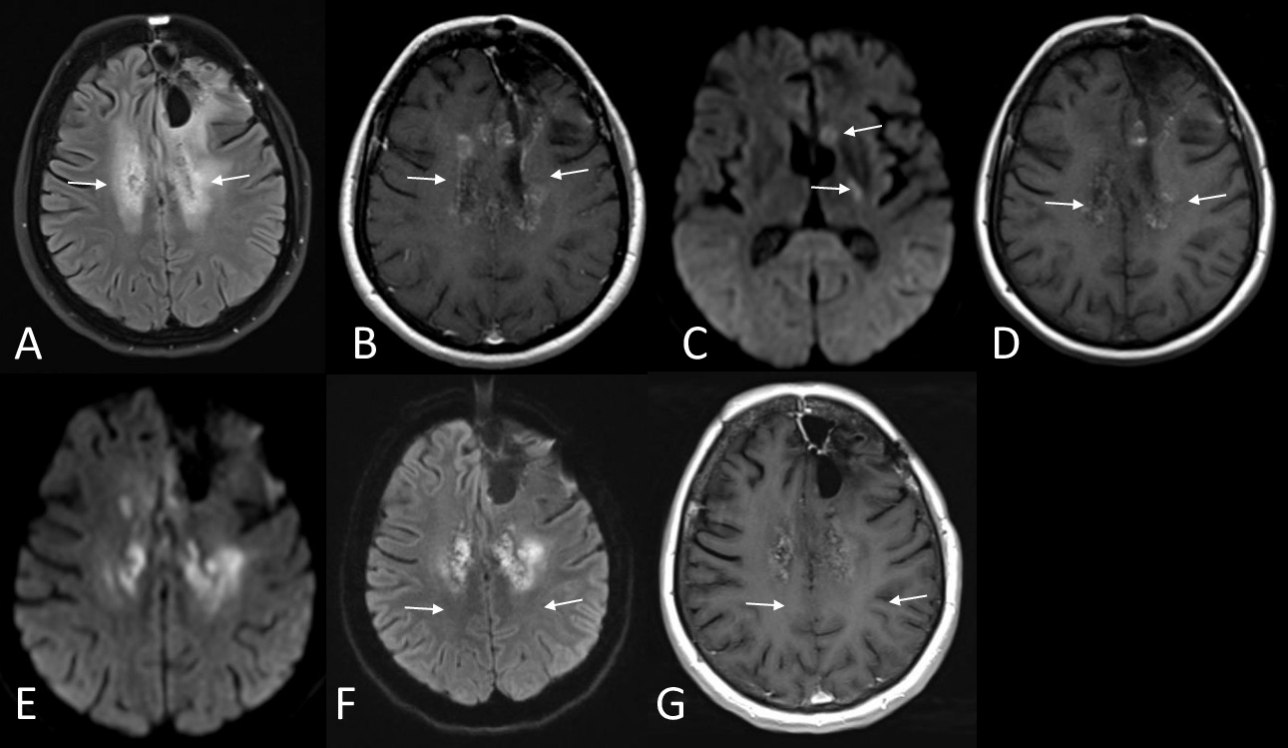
Patient with recurrent glioblastoma, treated with bevacizumab as mono-therapy, shows diffuse oedema in the *T*_2_ FLAIR images (arrows in A) surrounding the surgical cavity. The post-contrast *T*_1_WI show patchy, confluent enhancement in the area with abnormal *T*_2_ signal intensity (arrows in B) and a few areas with restricted diffusion (arrows in C). 6 months later, the gadolinium enhancement has decreased (arrows in D) suggesting hence response. The diffusion restriction (arrows in E) appears to be increasing in the areas of the previous patchy enhancement and the DWI retains its high signal 6 months later (arrows in F) confirming partial response to therapy. The contrast enhancement has also stable appearances (G). The diffusion restriction is believed to represent ischaemic tissue with coagulative necrosis. DWI, diffusion-weighted imaging; FLAIR, fluid attenuation inversion recovery; *T*_1_WI, *T*_1_ weighted imaging..
